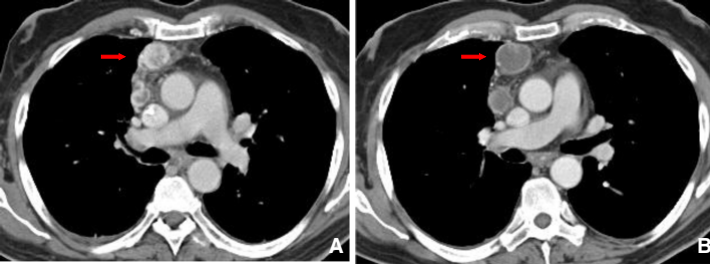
68 year old male with metastatic thyroid cancer. CT scan with contrast (Figure A) shows hyper vascular mediastinal nodes (arrow) consistent with metastatic disease. CT scan obtained after 2 cycles of Gefitinib (Figure B) demonstrates decrease in the attenuation of mediastinal nodes (arrow) reflecting desvascularization, with a thin rim of residual peripheral enhancement.
